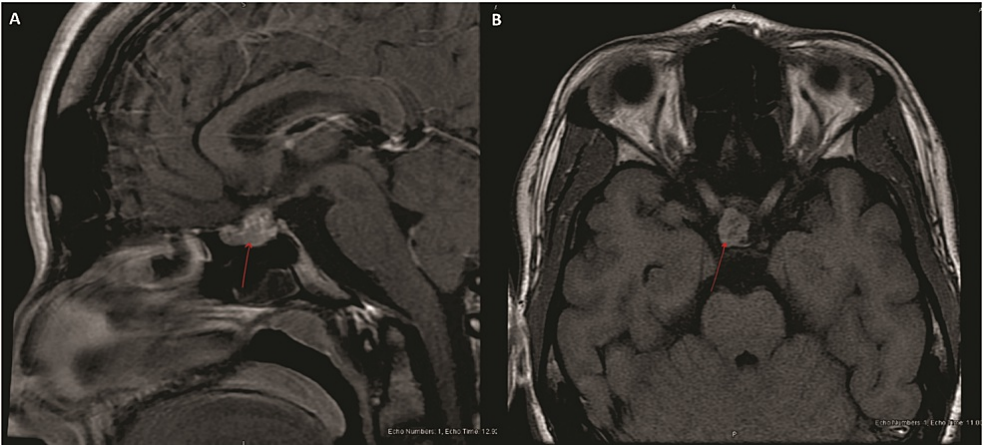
Sagittal and axial T1-weighted images Sagittal T1-weighted image showing a 1.9 x 1.3 x 1.3 cm lobular mass centered in the sella and suprasellar region (A). Axial T1-weighted image showing the mass (B).
